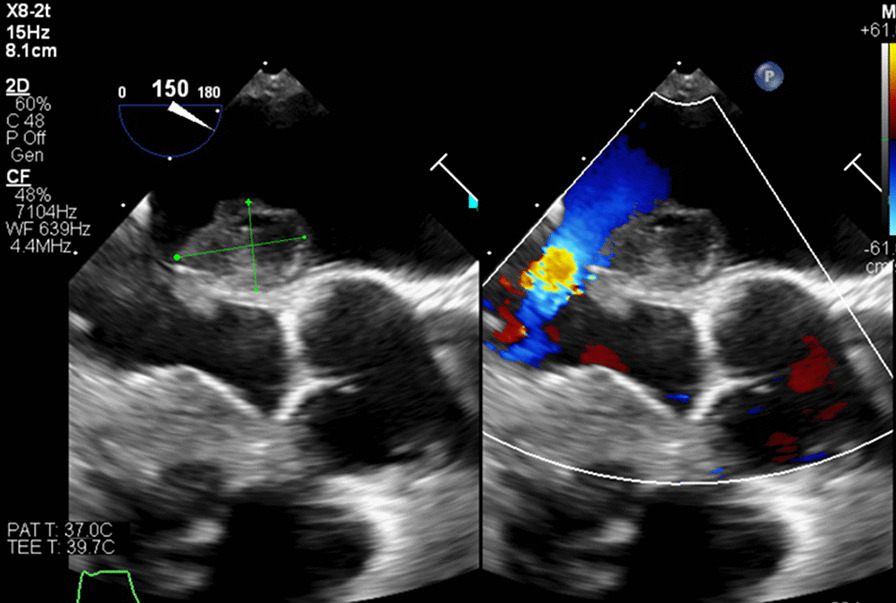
Transesophageal echocardiogram showing 1.9 cm × 1.3 cm polypoid echodensity on the atrial side of the anterior MVL/along the aortomitral cushion
